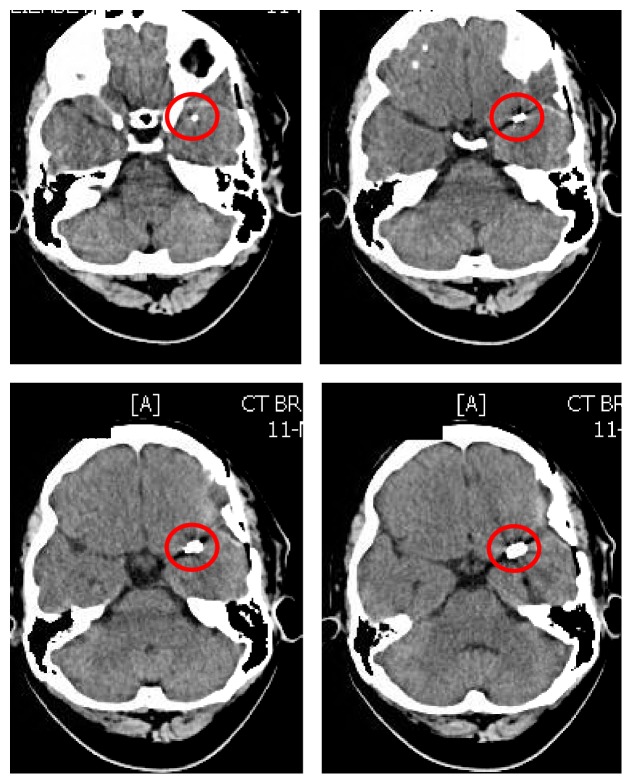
CT scan of brain without contrast axial view on November 11, 2016, after left temporal craniotomy and clipping of left MCA aneurysm: showing the clip in the area of the proximal left middle cerebral artery (red circles).
